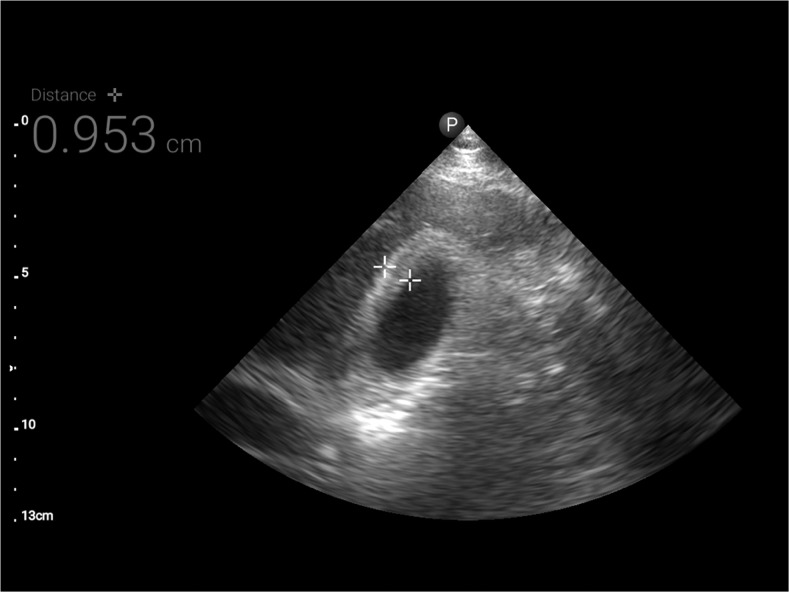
Ultrasound image showing gall bladder wall thickening of 0.93 cm (normal < 0.3 cm).
